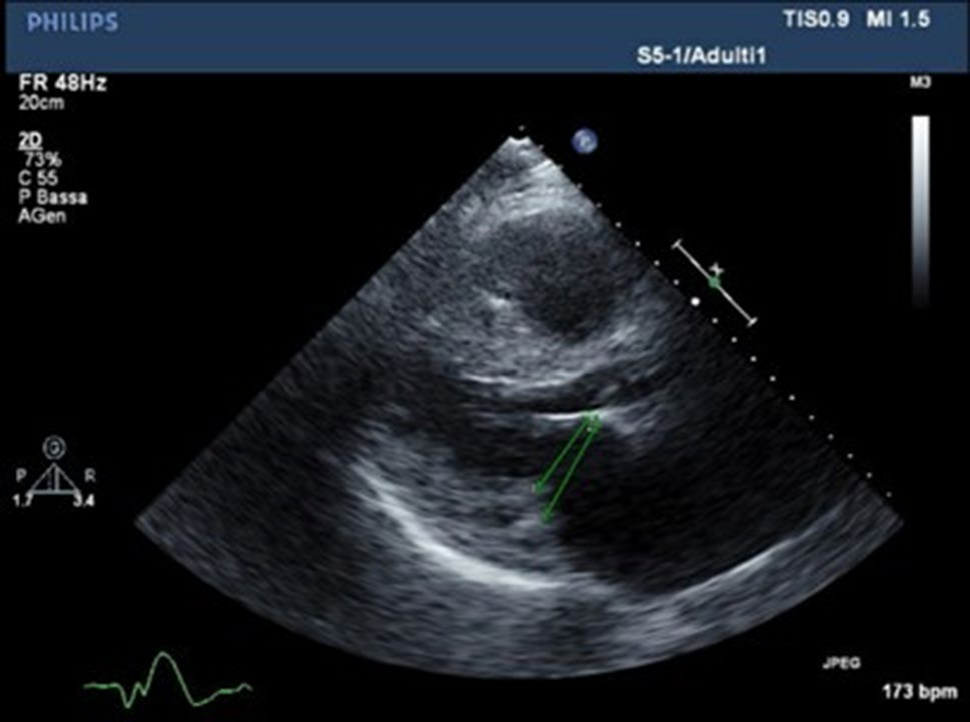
Standard and modified anteroposterior diameter of the mitral valve annulus in a in a 75-year-old man with cardiac transthyretin amyloidosis. Parasternal long-axis view. The modified diameter of the mitral valve annulus is marked as 1, while the standard diameter as 2. See text for further details
